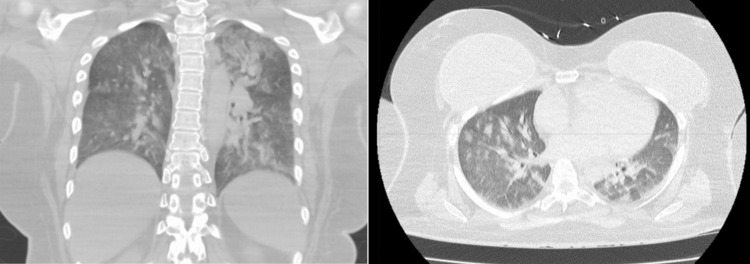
CT chest showing diffuse, bilateral ground-glass and consolidative opacities, peri broncho vascular wall thickening, and tree-in-bud opacities.
